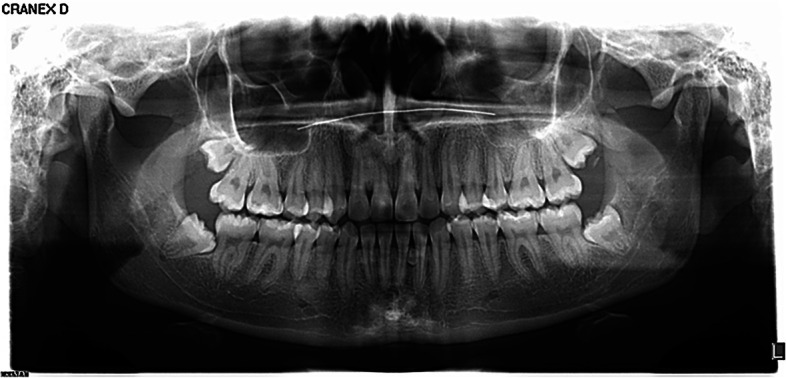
Panoramic radiograph number 3 used in the survey for evaluation of MTM 38
